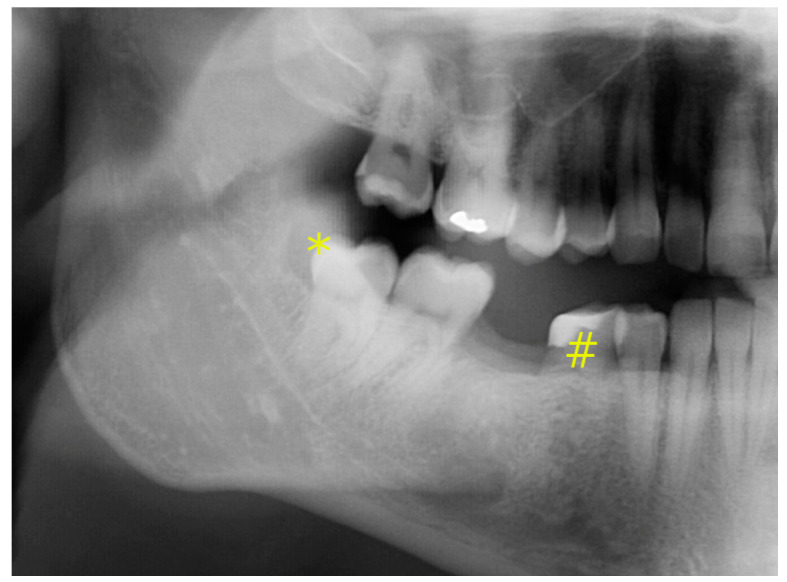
Initial panoramic radiograph; * Signs for pericoronitis tooth 48 as indication for tooth removal; # Deciduous tooth 85 with heavy restoration and severely resorbed roots and a poor long-term prognosis.
